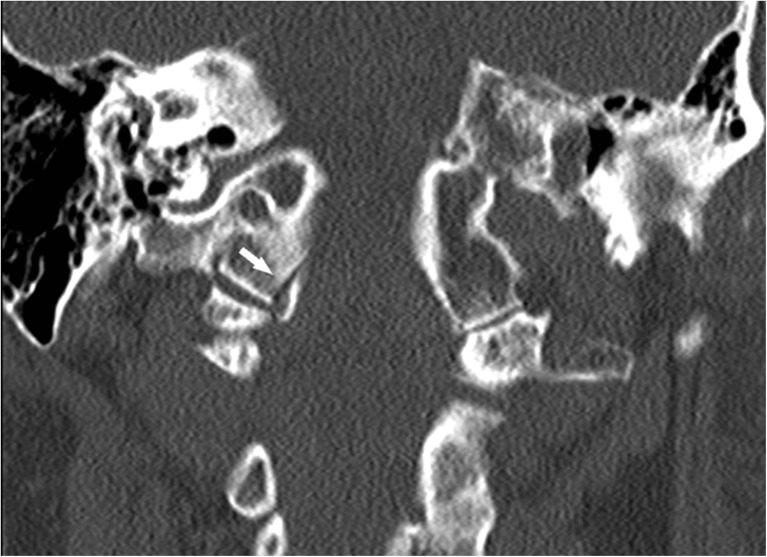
Coronal CT reconstruction of the craniocervical junction demonstrating an avulsion fracture of the right occipital condyl with inferomedial displacement of the fractured fragment (*white arrow*). The fracture is classified as an OCF type III according to Anderson and Montensano [1]
